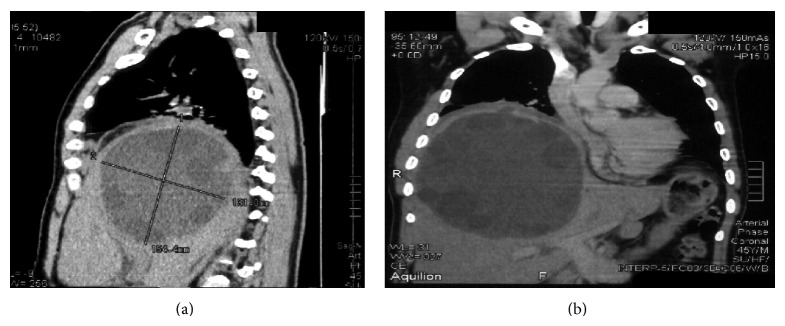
CT scan of large liver hydatid cysts < 12 cm with significant elevation of the right diaphragm.
